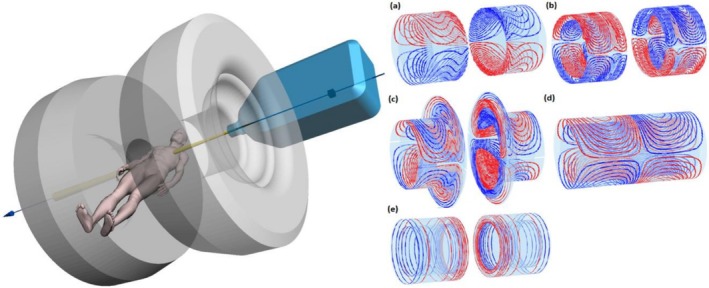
Australian MRI linac concept design (left) [14] (with permission) and field designs for the Australian MRI linac to incorporate the large split between the two halves of the device (right).
